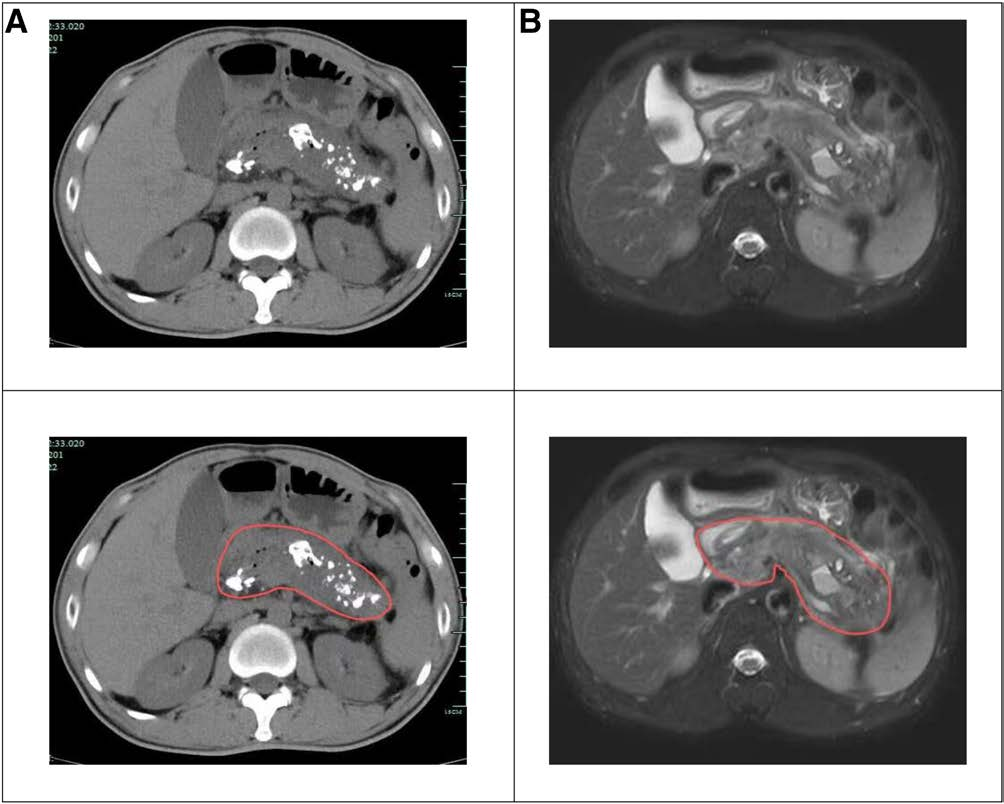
CT and MRI findings of the patient. (A) CT reveals pancreatic swelling with multiple dense foci and minimal gas accumulation. Increased peripheral density is noted, along with mild exudative changes (indicated by the circle). Thickening of the left renal anterior fascia is observed. The pancreatic duct shows bead-like dilation, containing nodular dense shadows and a stent. (B) MRI findings post pancreatic duct stent placement include pancreatic swelling and marked ductal dilation. Multiple small nodular short T2 signal foci are seen within the lumen. The pancreas exhibits increased signal intensity on T2 fat-suppressed imaging, with areas of markedly high signal on DWI. Peripancreatic exudative changes are noted on DWI (indicated by the circle), accompanied by thickening of the left renal anterior fascia.
